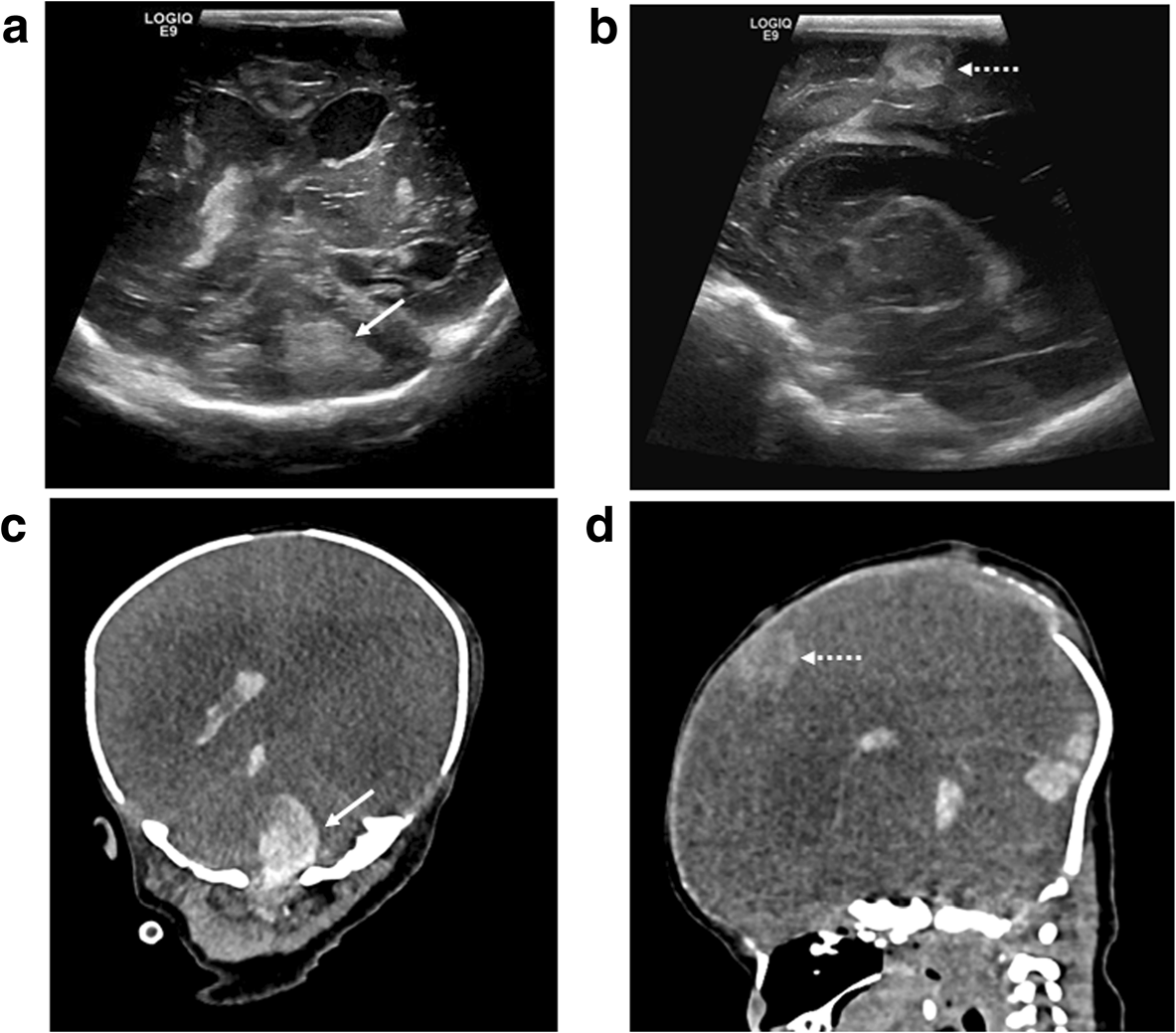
Post-mortem cranial ultrasound images (top row), with matched unenhanced post-mortem CT images (bottom row) of a fourteen day old term neonate who died from a metastatic fibrosarcoma affecting the right thigh. Both imaging modalities were performed 3 days after death. The coronal (a, c) and sagittal (b, d) images demonstrate bilateral lateral ventricular dilatation with several hyperechoic and hyperdense metastatic deposits within the cerebellum (white arrows) and along the falx (dashed arrow). There is loss of grey white matter differentiation with poorly defined ventricles on the CT compared to ultrasound, given the significant underlying cerebral oedema
